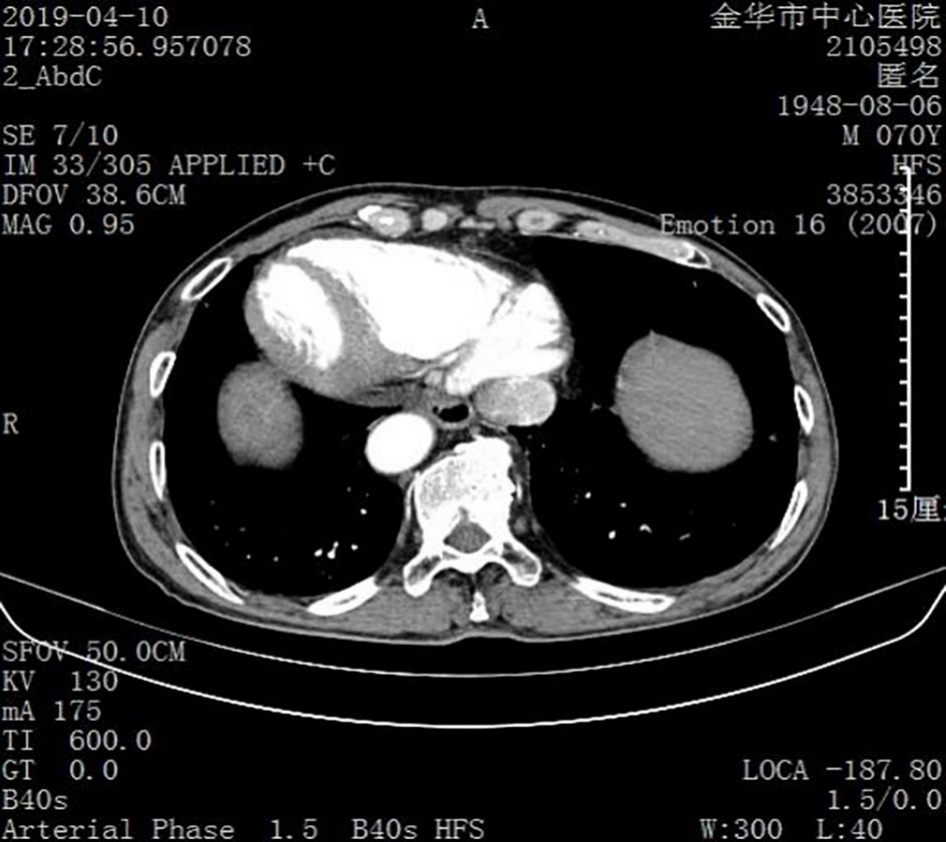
Image obtained using computed tomography. The organs are inverted.
